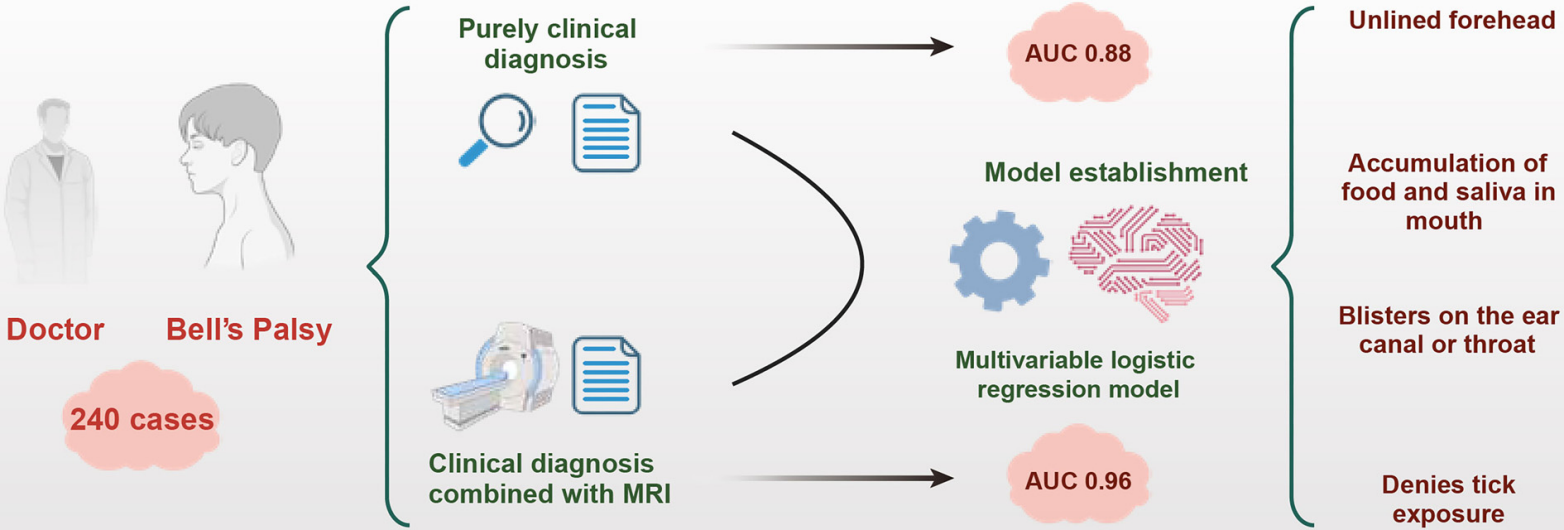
Development and validation mechanism of the primary medical diagnosis model for Bell’s palsy in primary healthcare institutions. AUC: Area under the curve; MRI: Magnetic resonance imaging.
